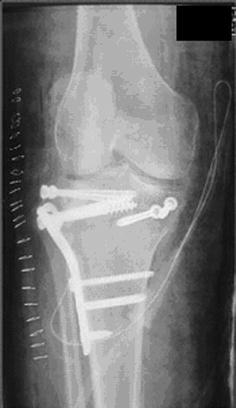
Schatzker type V fracture, post-op X-rays (AP)
